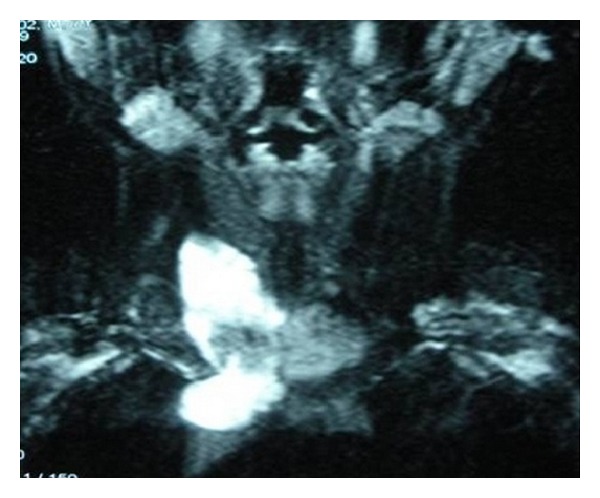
Magnetic resonance imaging of the cervical cyst disclosing its relation to the larynx.
